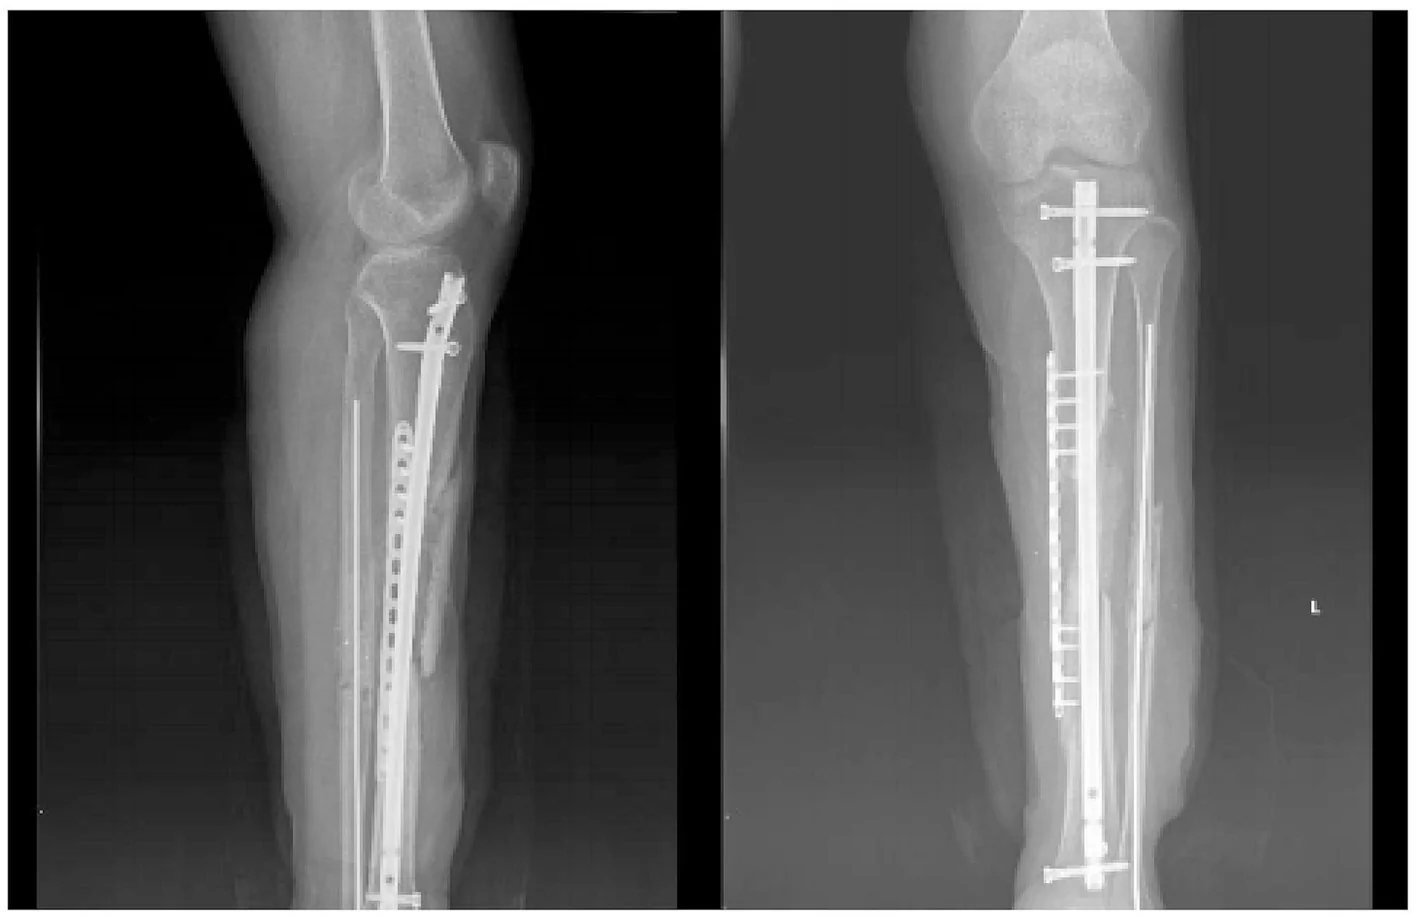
The immediate X-ray findings two days after the bone grafting procedure.
